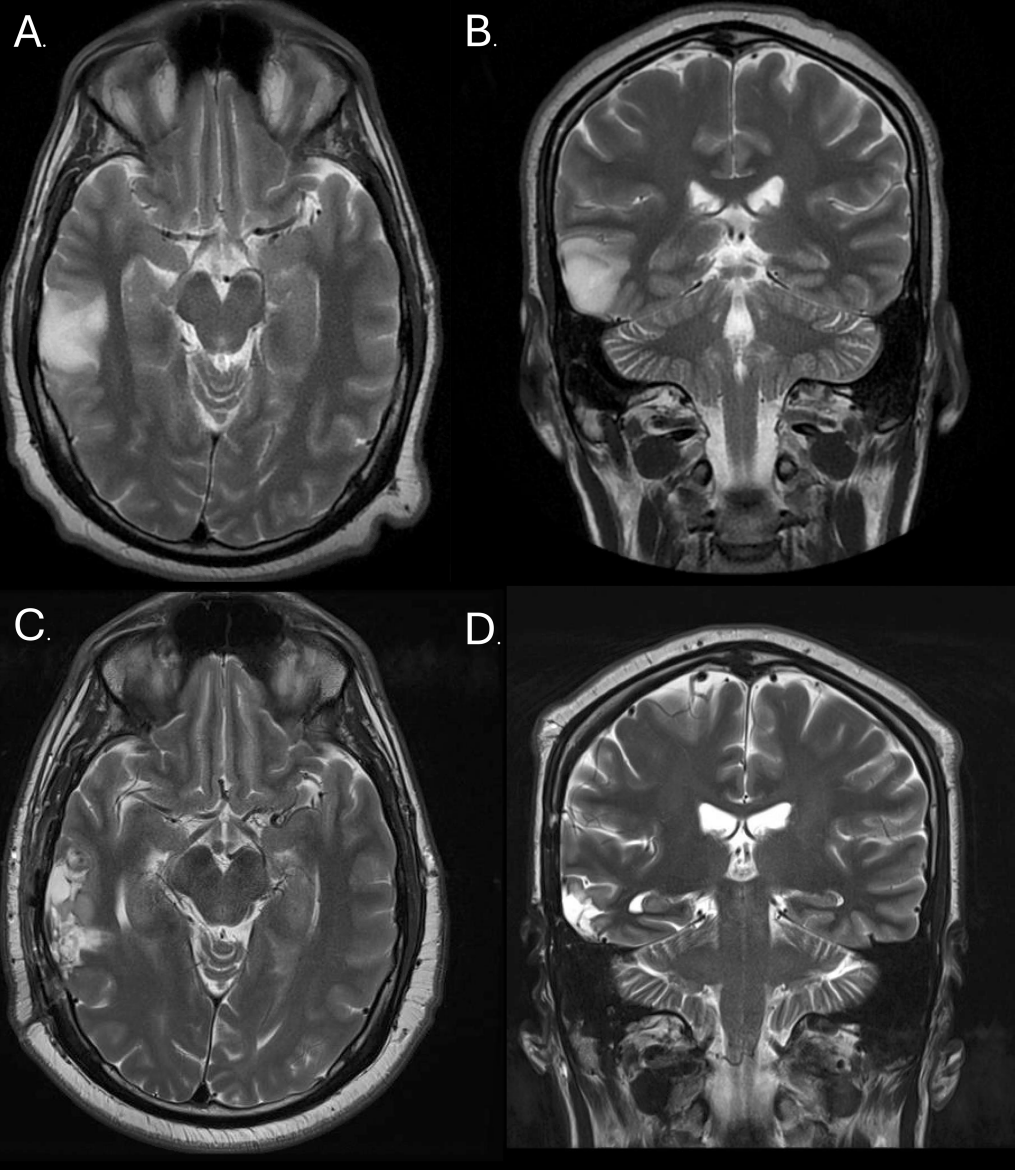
Right Temporal Astrocytoma: A T2-Weighted MRI image prior to resection (transverse (A) and frontal (B) planes) and post-resection (transverse (C) and frontal (D) planes).
